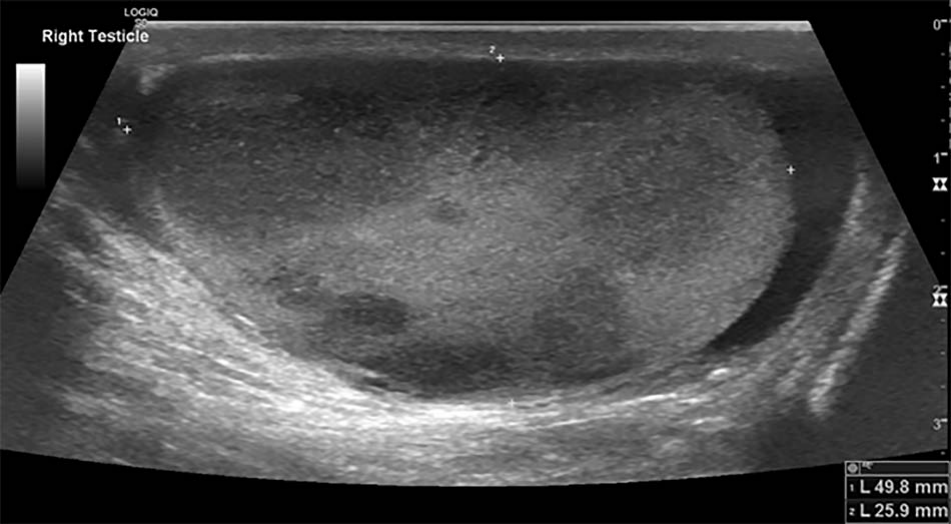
Testicular ultrasound of the right testicle demonstrating multiple hypoechoic lesions—the largest measuring 3 cm.
